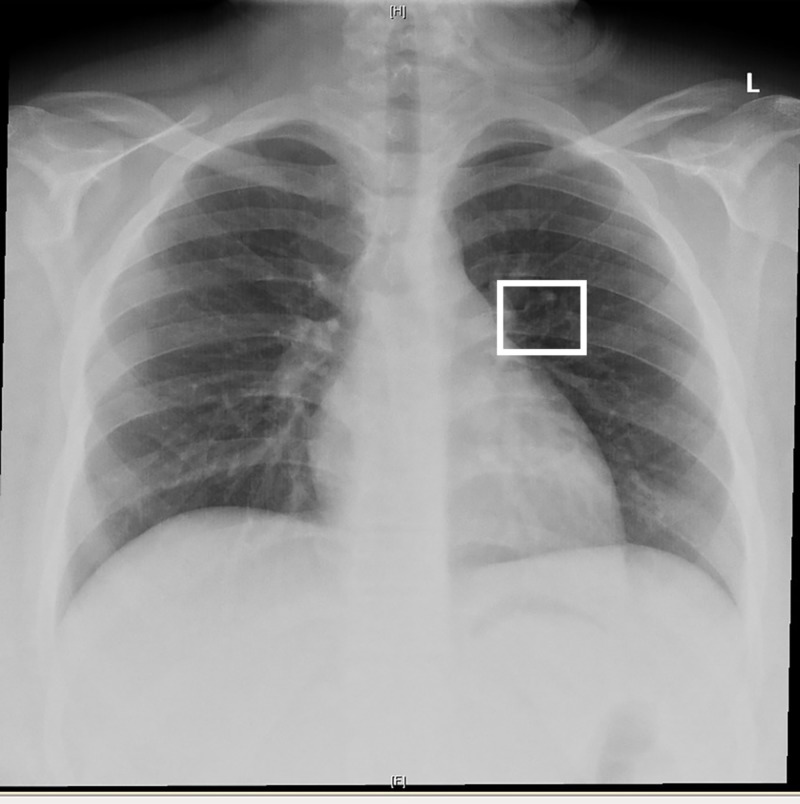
Anteroposterior view of chest X-ray. The anteroposterior view of a chest X-ray showed the presence of a bifid fifth rib on the left side (white box).
